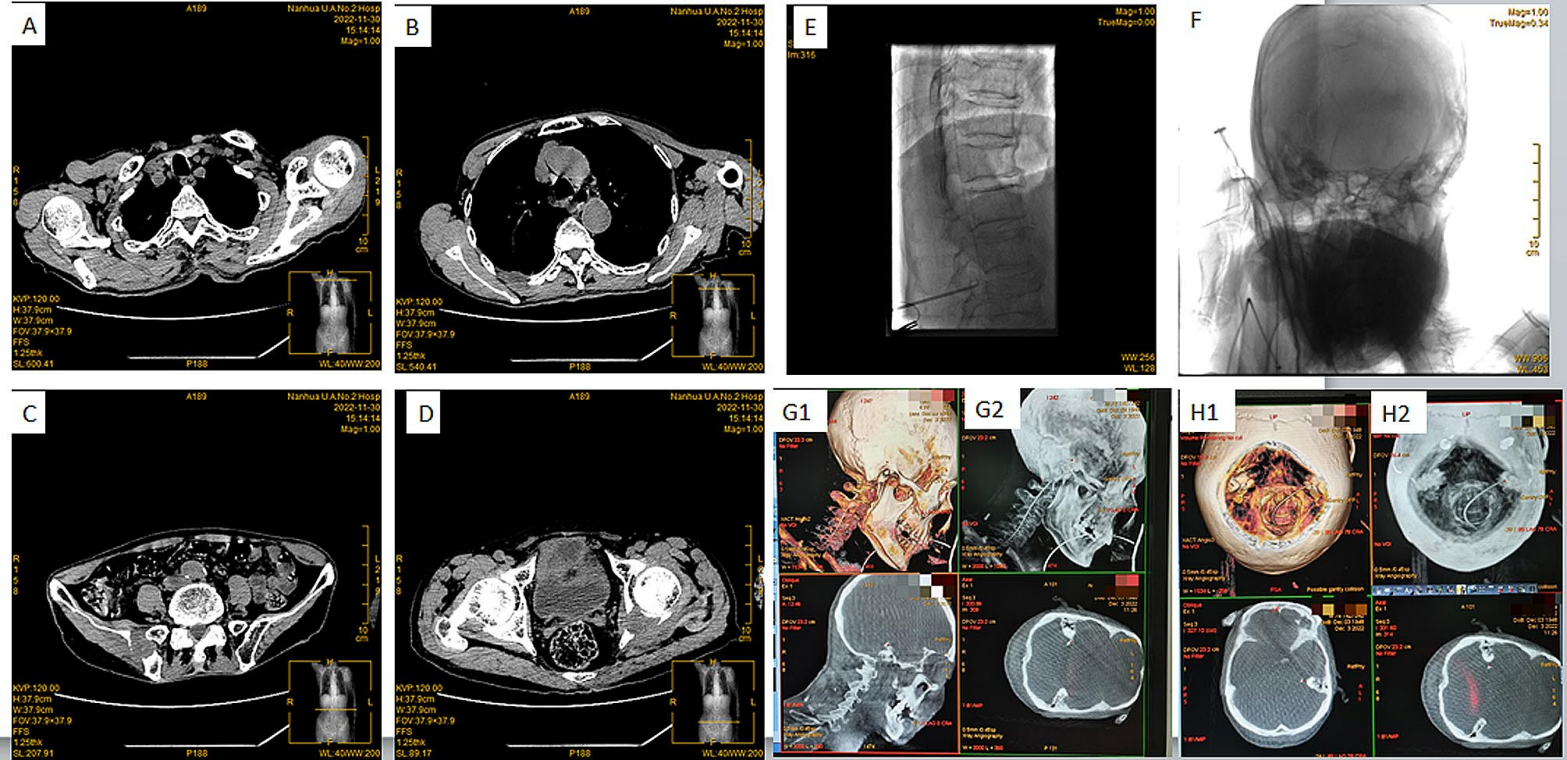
(A-D) The computed tomography images of the patient shows (A) the thickening of the esophageal wall (white arrow), (B) the rib bone lesion (blue circle), (C) the lesion on the right iliac crest (yellow circle), and (D) the lesion on the left ischium (red circle). The digital subtraction angiography shows (E) the catheter in the spinal canal, and (F) the catheter tip near the prepontine cistern. The 3D construction images shows the catheter (G1-2) and (H1-2) reconstruction of the skull base to the real location of the catheter
